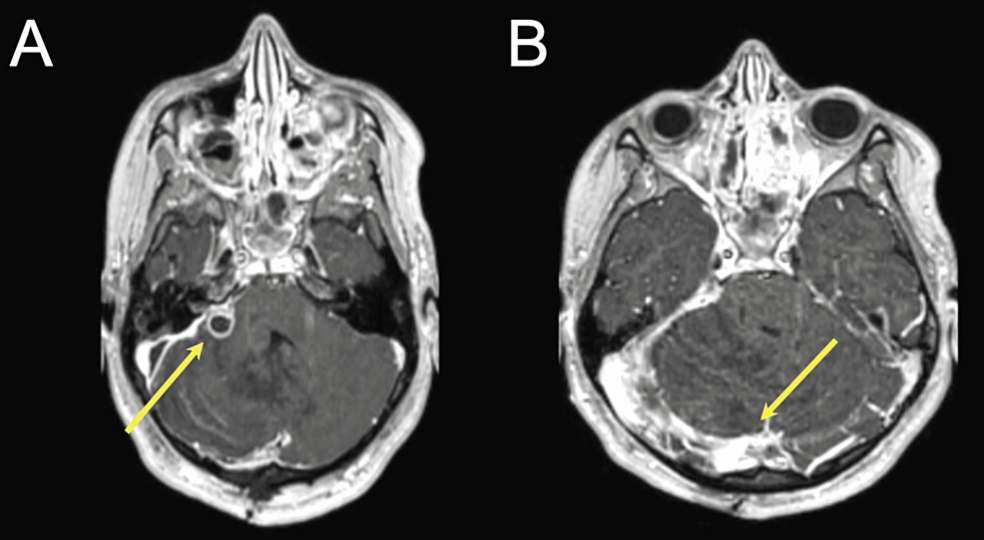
Contrast-enhanced T1-weighted MRI showed a small abscess in the right cerebellopontine angle (A) and thrombosis of the right transverse sinus (B).
